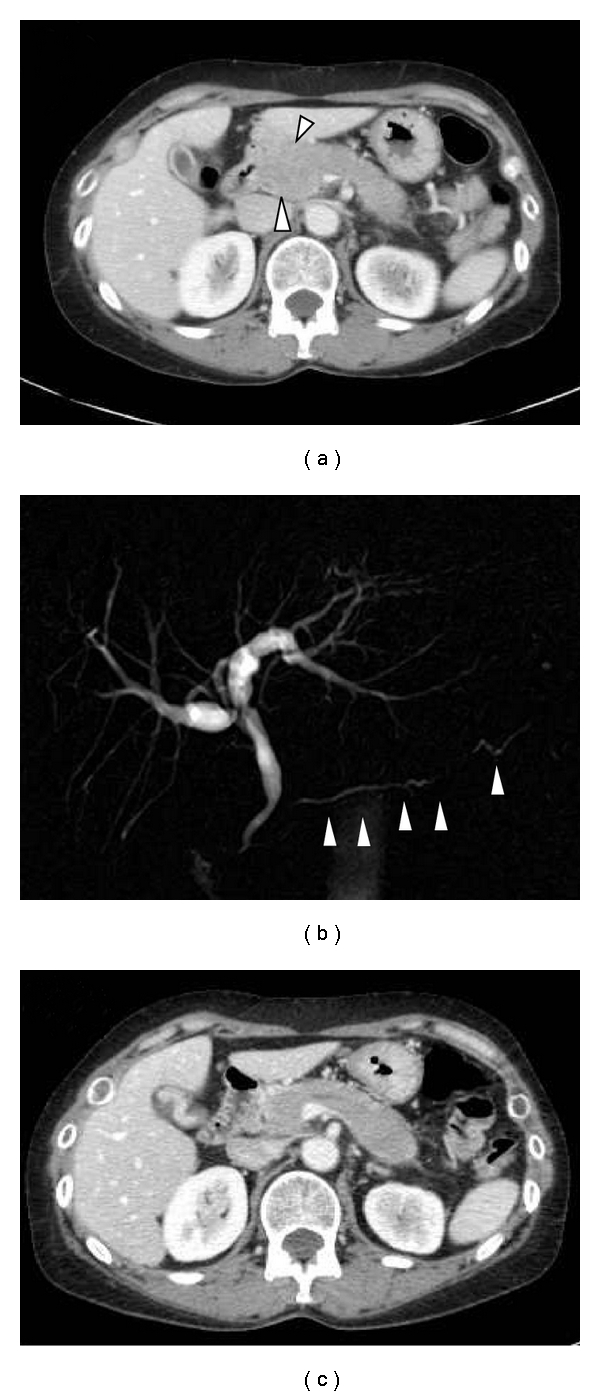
(a) CT scan of the upper abdomen. Arrowheads indicate swollen pancreas. (b) MRCP of the abdomen. Arrowheads indicate pancreatic duct. (c) CT scan after administration of prednisolone.
